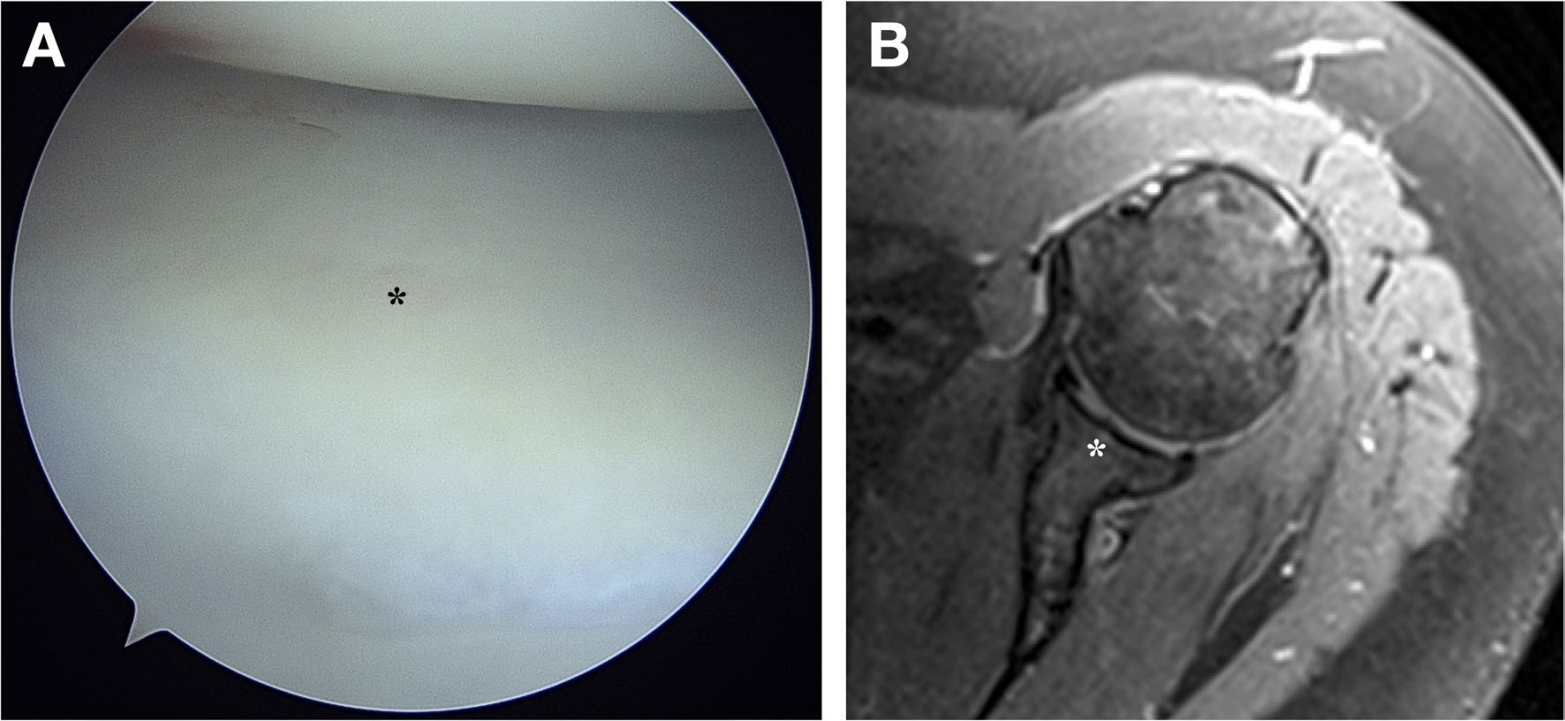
Arthroscopic view on a centrally located greyish area (asterisk) corresponding to the zone of thinned articular cartilage (A) and the MRI showing the underlaying tubercle of Assaky (asterisk) (B)
